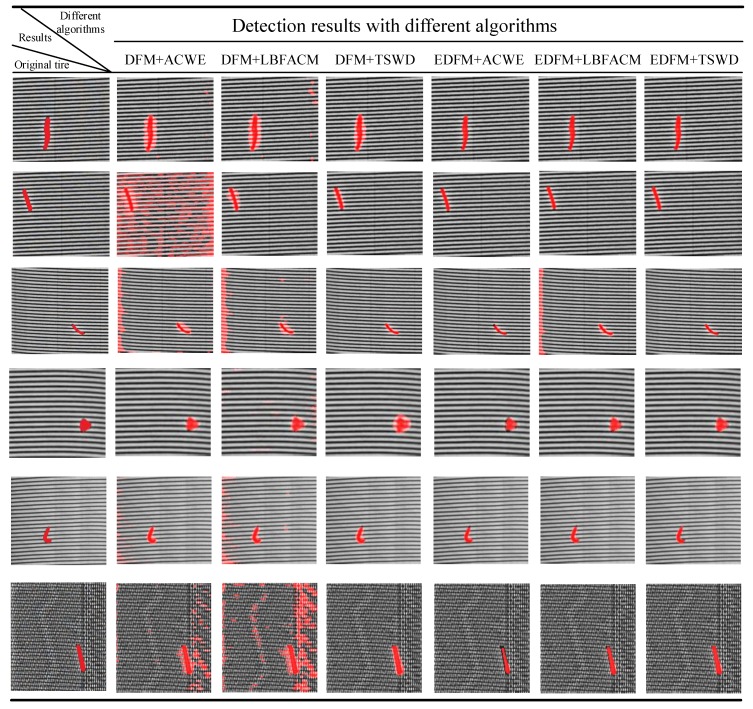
Detection results with different algorithms for tire X-ray images.
